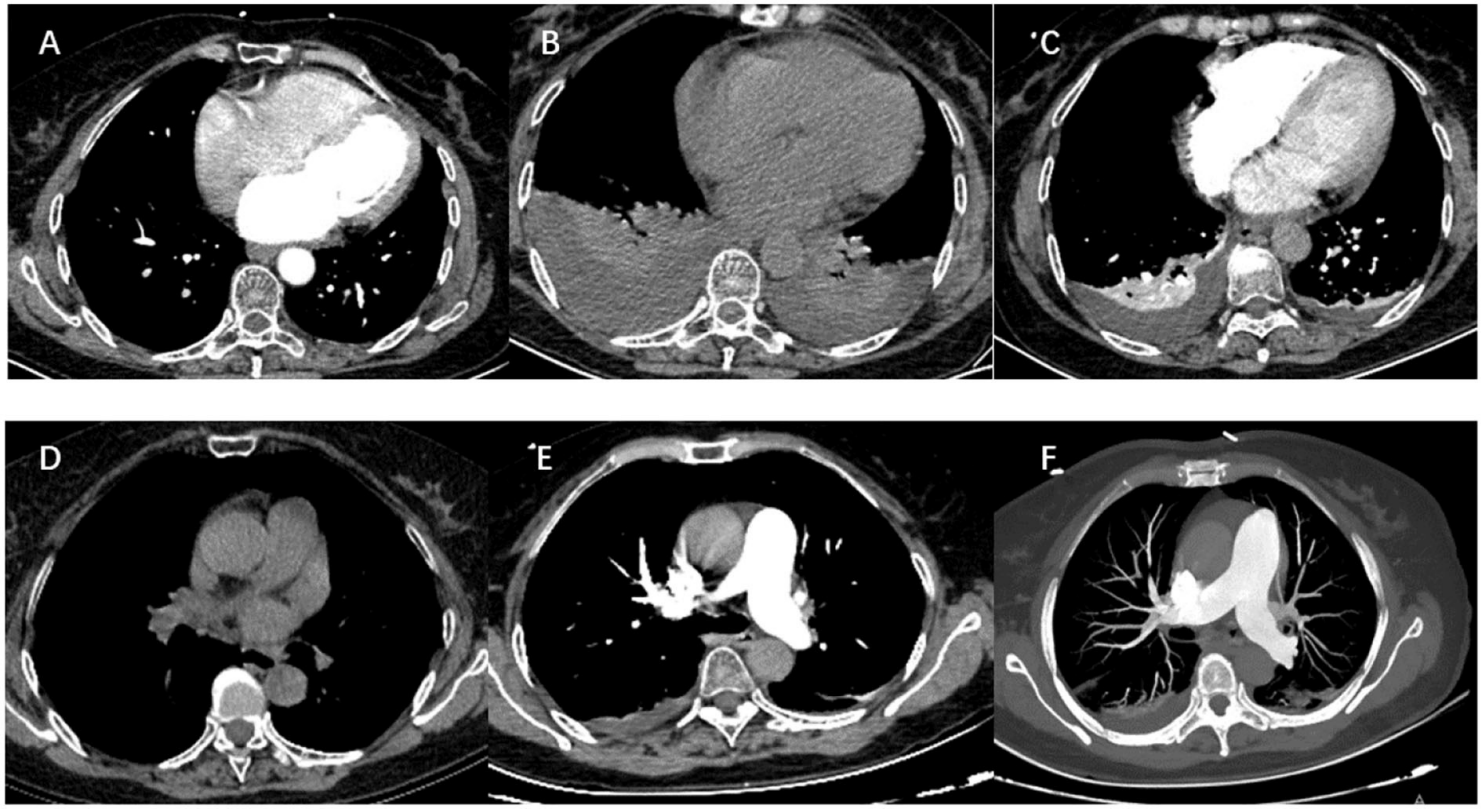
The dramatic transition of a thoracic CT scan (soft-tissue window) and pulmonary computed tomography angiography. (A) Preoperative tests: CT revealed clear lung fields bilaterally. (B) Three days after operation: CT scan showing large bilateral pleural effusions without pericardial effusion. (C) Nine days after the operation: there is a resolution of the left pleural effusion and a marked decrease in the right pleural effusion. (D) Nineteen days after operation: there is no pleural effusion on CT images. (E,F) Pulmonary computed tomography angiography ruled out pulmonary embolism.
